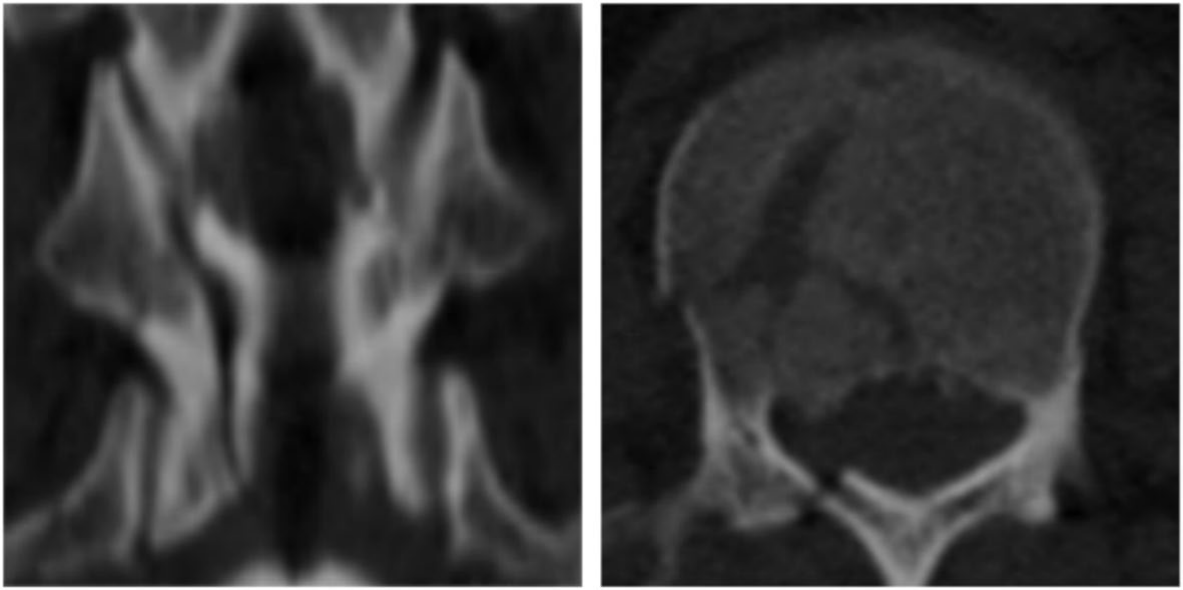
Complete laminar fractures. CT axial and coronal planes show the fracture line through all lamina
